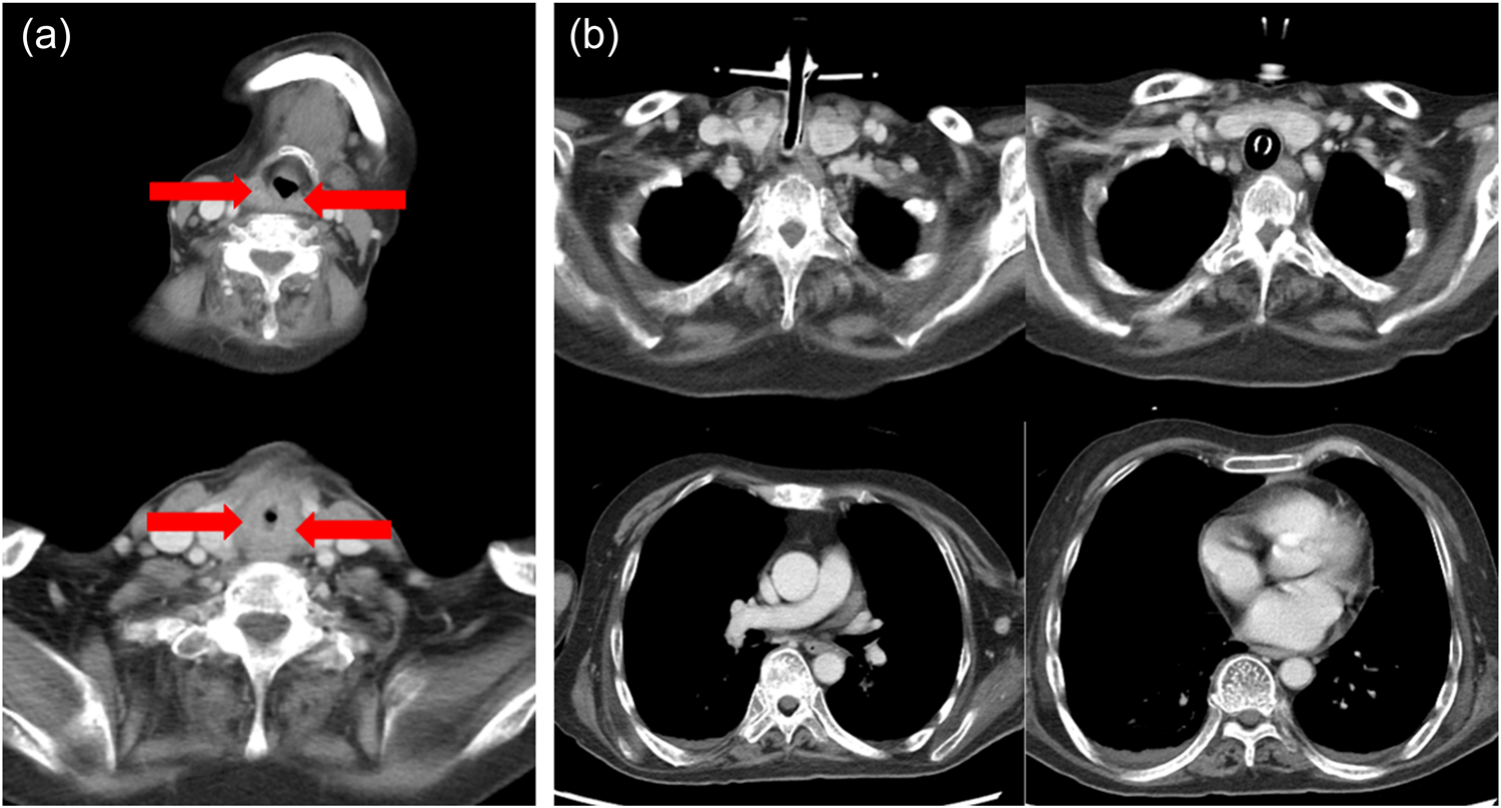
Contrast‐enhanced computed tomography. (a) The nasogastric tube is removed. Marked edema is observed in the arytenoid region, as indicated by the red arrow. (b) No structural abnormalities are identified from the neck to the chest that could explain the bilateral vocal fold paralysis.
